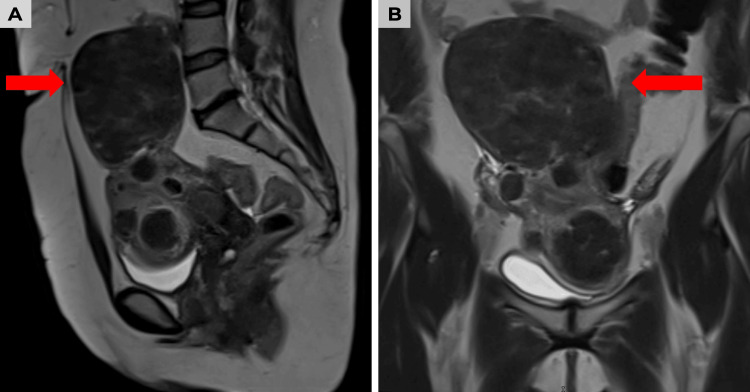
Abdominal-pelvic magnetic resonance imaging in T2 sequence showing an enlarged uterus with dominant myomatous lesion (arrows) (A) Sagittal section with a large myoma dependent on the uterine fundus. (B) Coronal section confirming the dominant lesion with an intramural/subserosal component and mass effect on pelvic structures.
